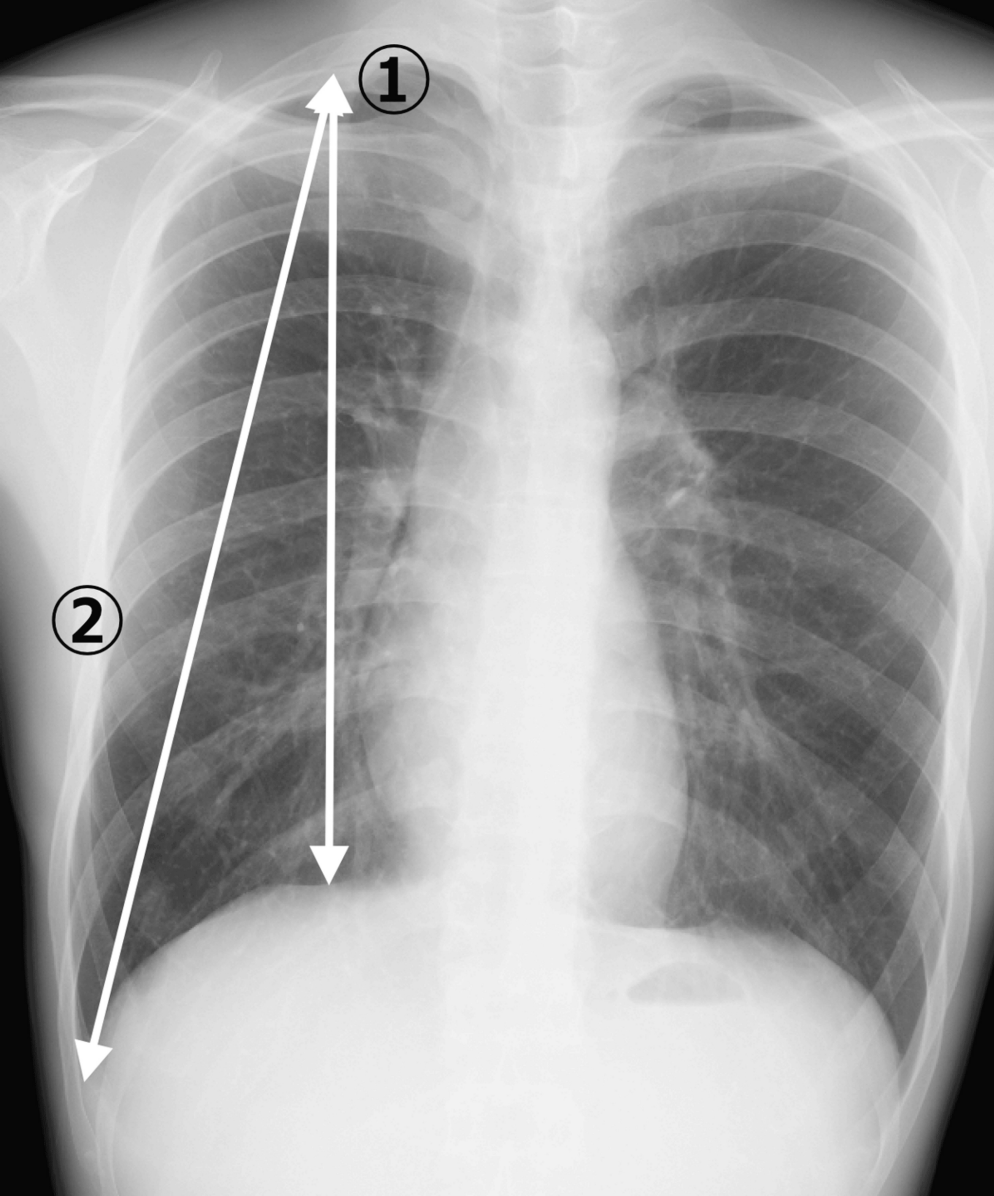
Vertical thoracic length measurement The length from the apex of the thorax to just above the diaphragm ① and from the apex of the thorax to the diaphragmatic angle of the ribs ② were measured using simple chest X-rays
